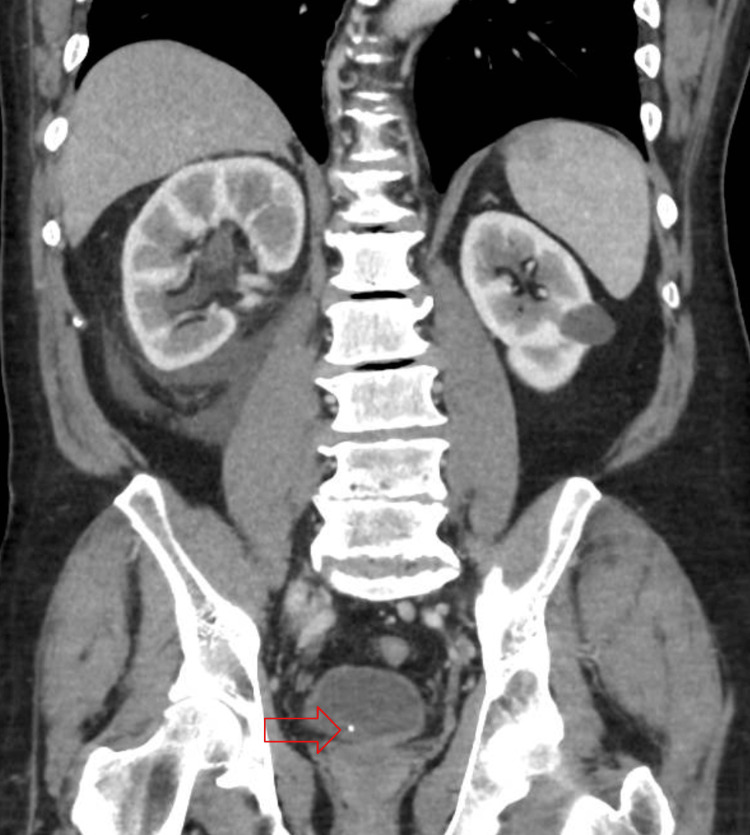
A coronal view depicting a stone measuring 3 × 2 × 2 mm visualized in the right ureterovesical junction (red arrow) on the post-contrast computed tomography scan.
